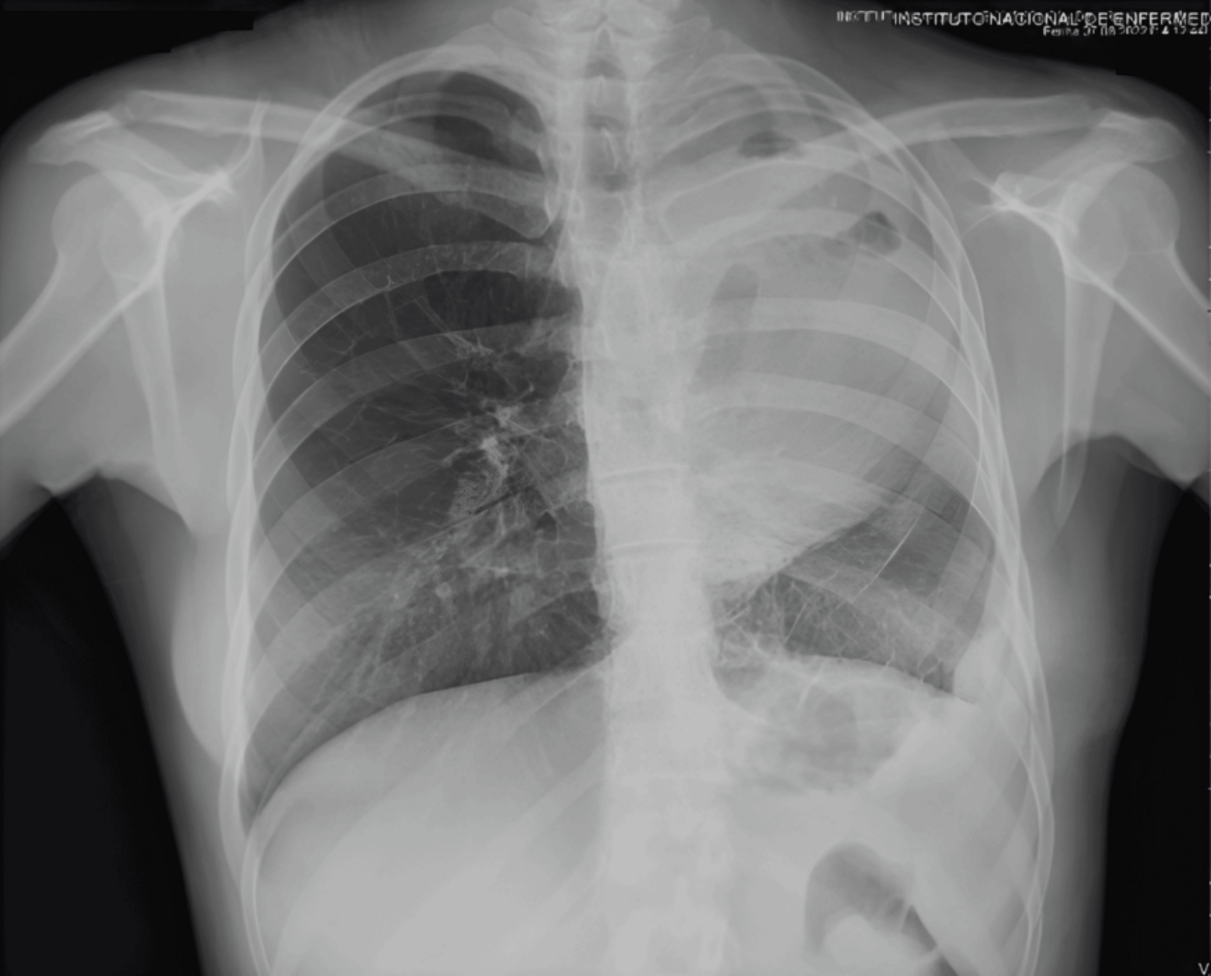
Postoperative chest X-ray
